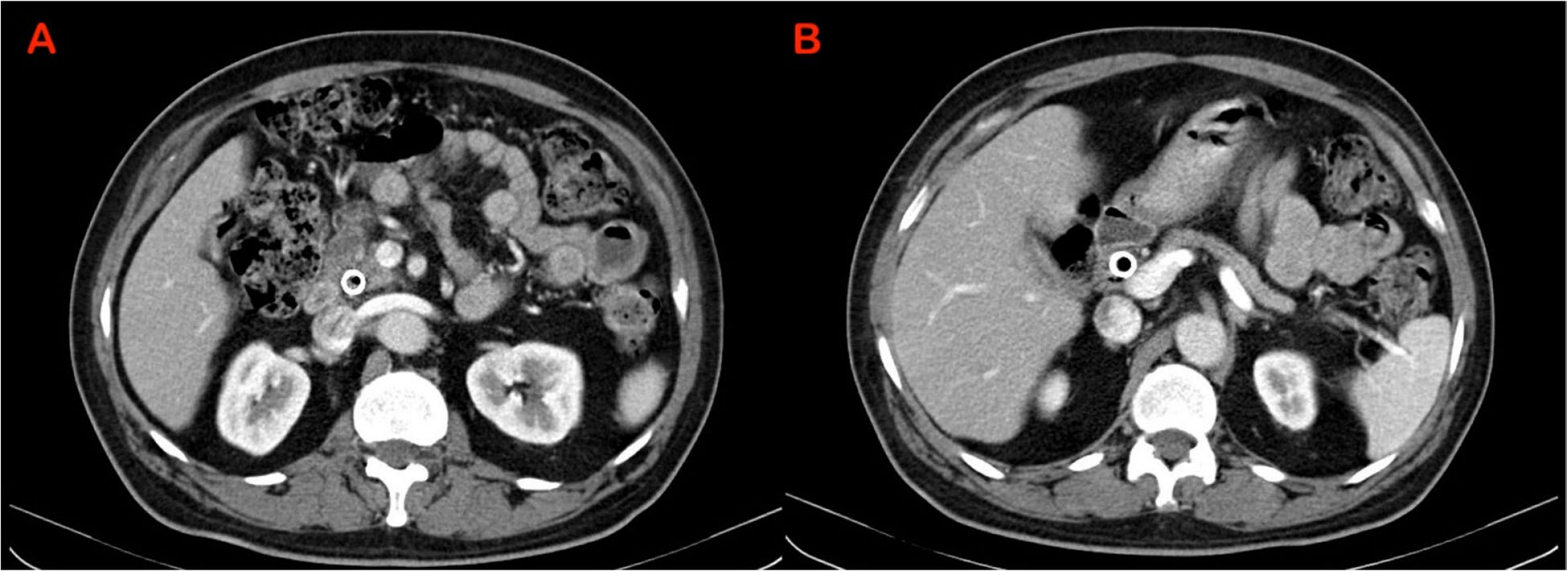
Computerised tomography (CT) shows post steroid treatment change in a patient with autoimmune pancreatitis (A) atrophy of the head of pancreas with a covered metal biliary stent in situ (B) atrophy of the body and tail of pancreas with undilated MPD
